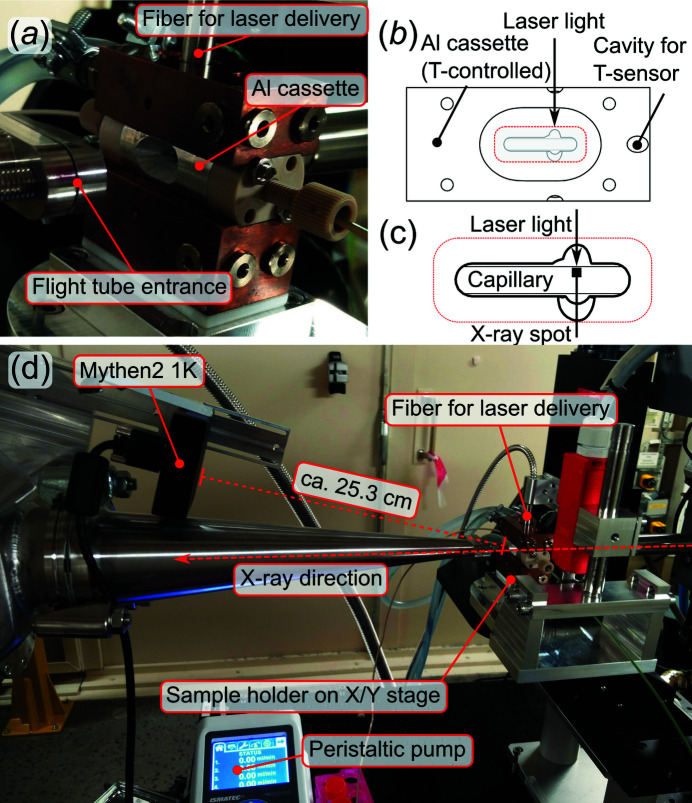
Flow cell and environment at the CoSAXS experimental table. (*a*) The flow cell capillary holder. (*b*) The aluminium cassette of the capillary holder and (*c*) a close up indicating where the X-rays and laser are directed. The size of the laser spot is ∼0.9 mm FWHM and the X-ray spot is ∼160 µm × 140 µm. (*d*) The setup at the sample table. Fresh sample is delivered through PEEK tubing by a peristaltic pump. The irradiating laser light arrives through a fiber. The sample holder is placed on an *X*/*Y* stage ∼25 cm from the WAXS 1D detector and 2 m from the 2D SAXS detector (outside photograph).
